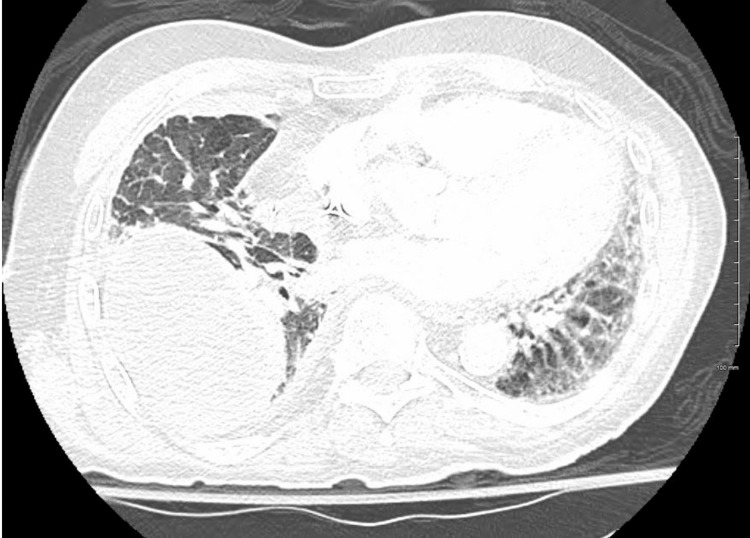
Chest CT (2nd Admission)
